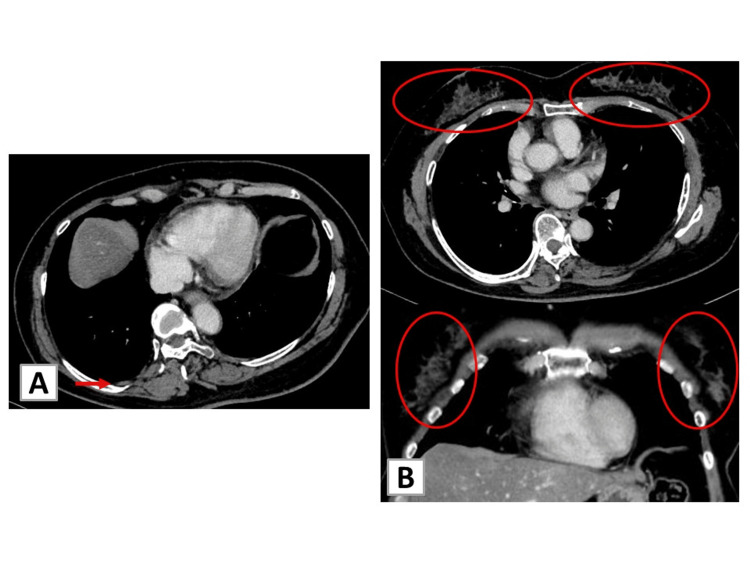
Chest CT findings at six months after the ALK–TKI treatment (A) CT showing tumor size reduction in the lower right lobe (arrow) and resolution of the pleural effusion. (B) CT in axial (upper) and coronal (bottom) views showing resolution of shadows in bilateral breasts (red circles). ALK, anaplastic lymphoma kinase; TKI, tyrosine kinase inhibitor
